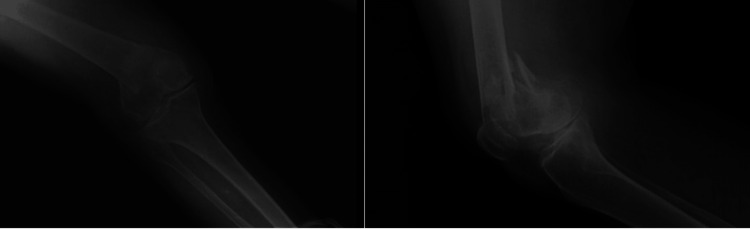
Case 3 preoperative X-rays: anteroposterior and lateral views
